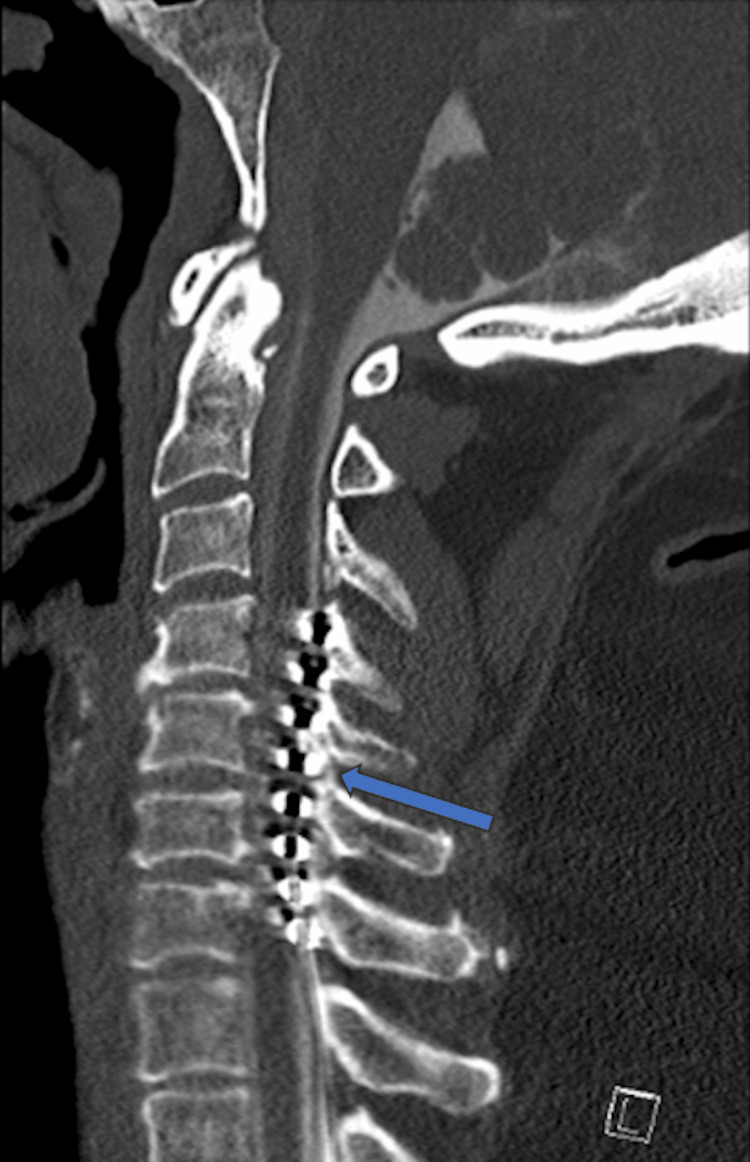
Preoperative sagittal CT scan of the cervical spine re-demonstrating spinal cord stimulator (SCS) leads in the cervical spine. The blue arrow denotes the presence of the SCS leads in the dorsal aspect of the spinal cord canal.
